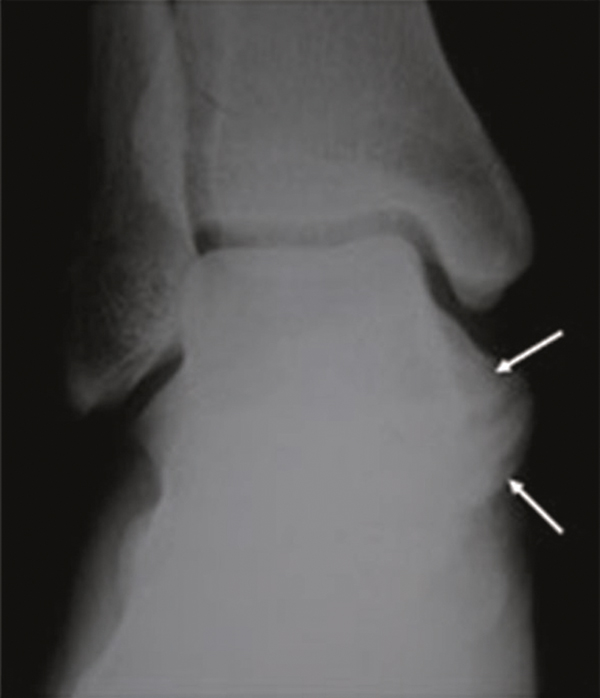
A preoperative radiograph of the foot shows a bony prominence (arrows) raising from posteromedial of the calcaneus.
